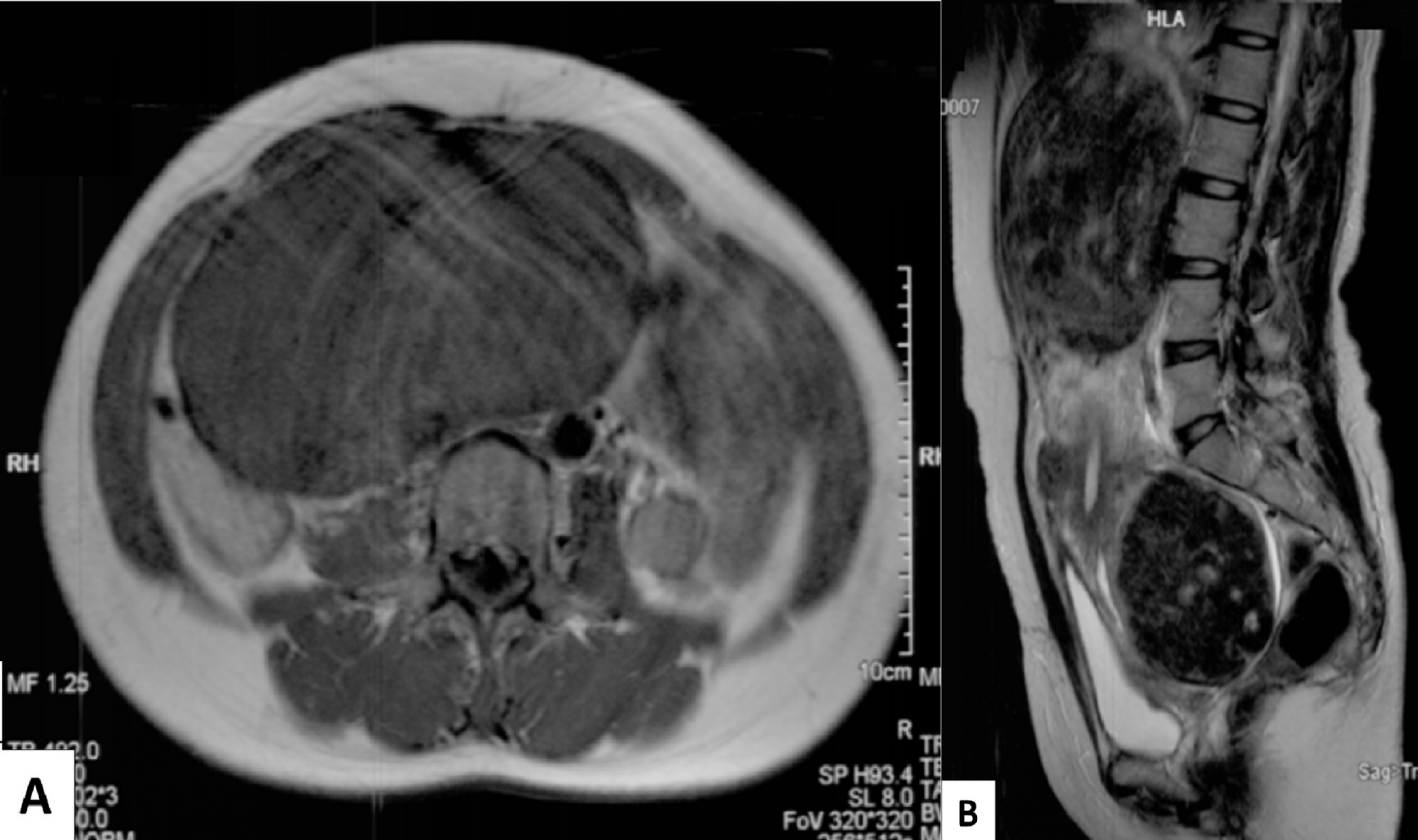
MRI before uterine artery embolization. (A) Axial image shows massive leiomyoma of intermediate signal intensity on T1-weighted image. (B) Sagittal T2-weighted image. Enlarged uterus compressed and displaced the bladder and bowel loops.
